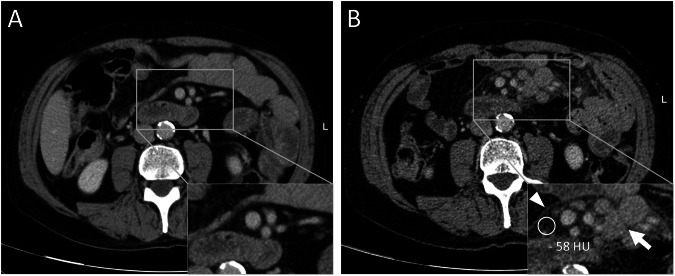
Abdominal CT images of a 71-year-old patient before (A) and 340 days after therapy start with Ipilimumab/Nivolumab showing newly developed mesenteric lymph node metastases (B). Arrowhead indicates pseudo-capsule, arrow points at mesenteric nodules and circular ROI shows increased density of the mesenteric fat. HU, Hounsfield units
